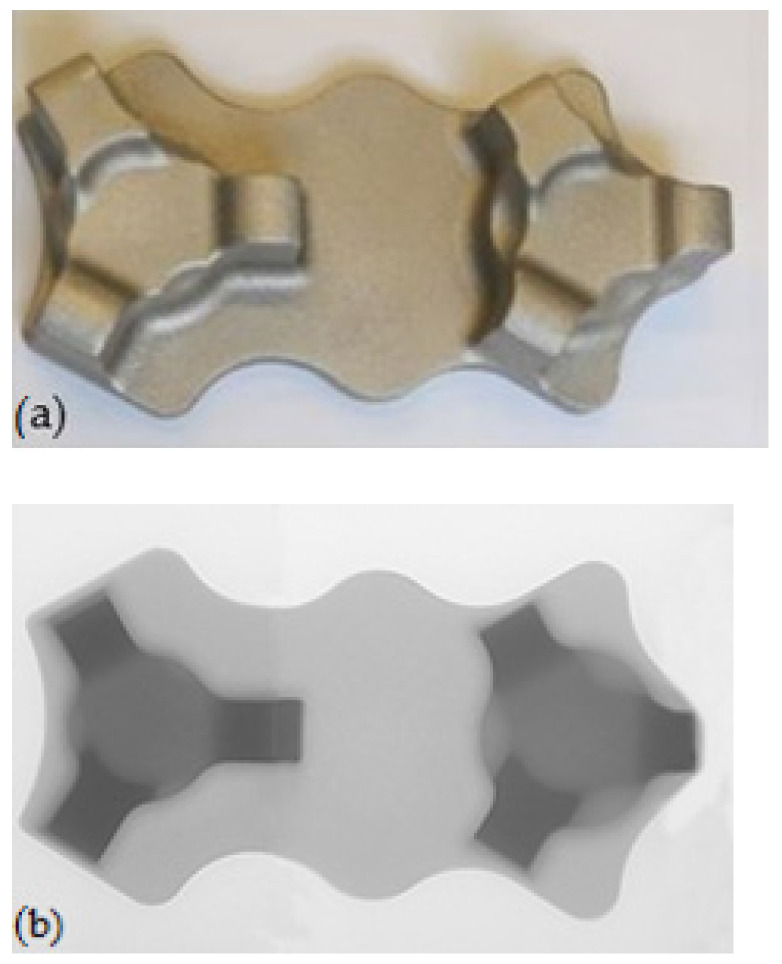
Cast preforms: (a) lower degree of forging, (b) X-ray of a preform.
